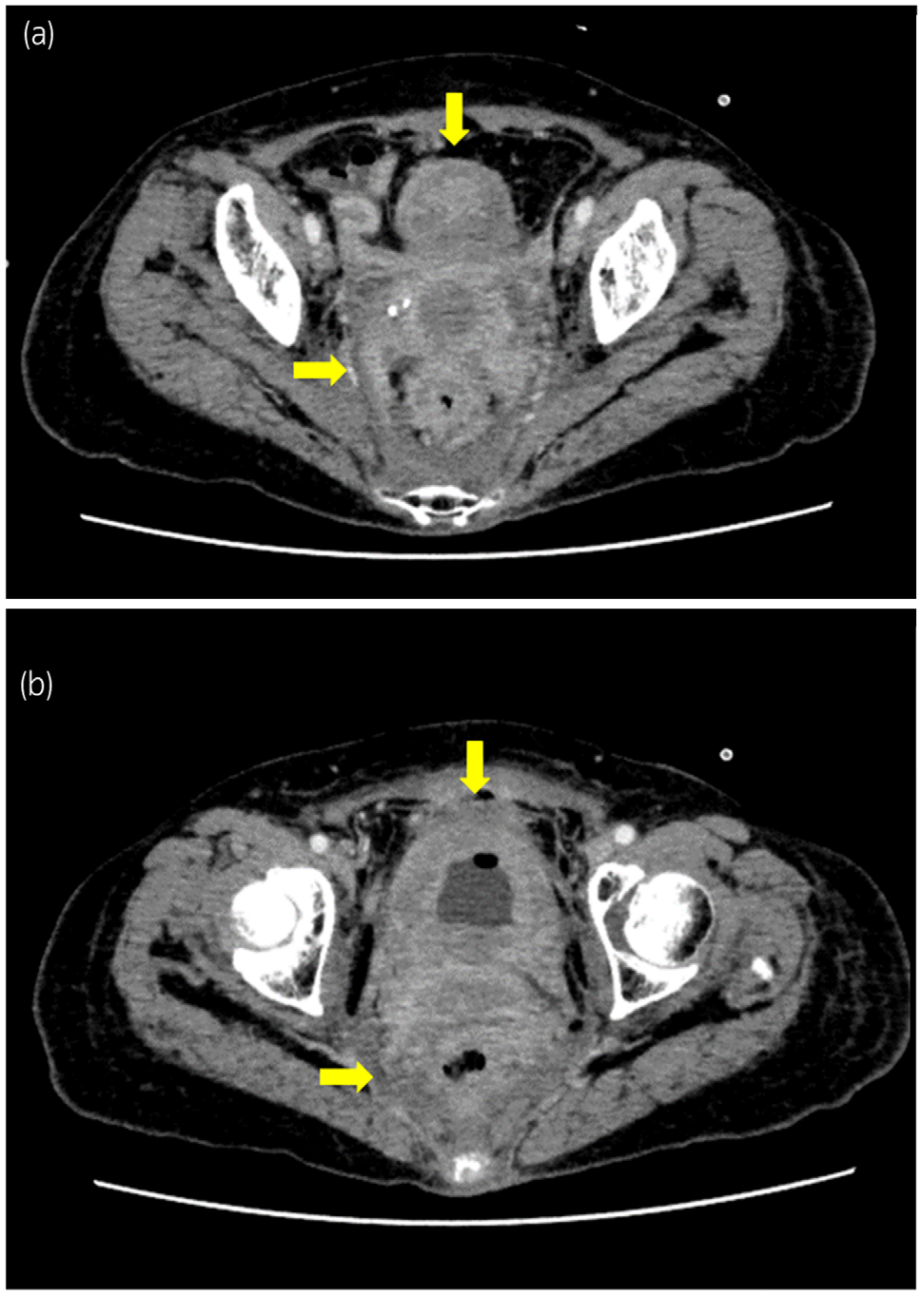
CT scan findings of the current case. (a) Ill‐defined soft tissues spread into the pelvis (arrows), which indicated retroperitoneal dissemination. (b) Diffuse thickening was observed in the bladder and rectum wall (arrows).
